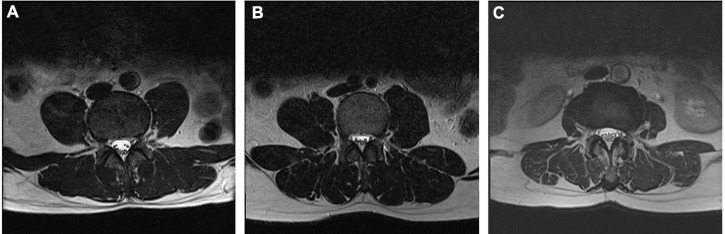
Representative MRI images used for Weishaupt grading system of lumbar facet joint degeneration. Axial T2-weighted views showing grade 1 (A), grade 2 (B), and grade 3 (C) osteoarthritis of the lumbar facet joint.
